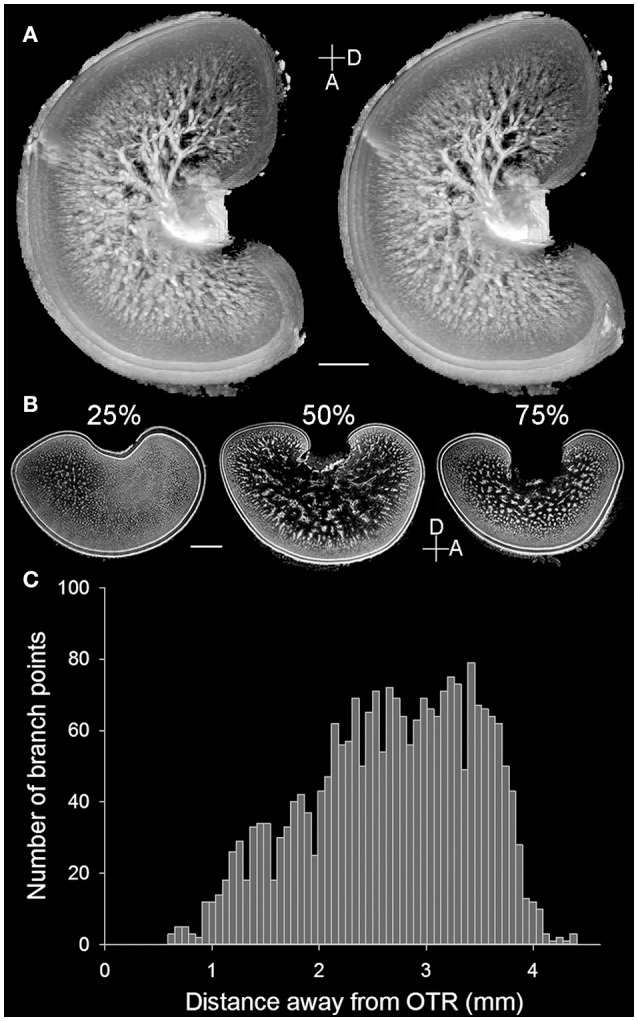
Cell islands in the medulla of the optic lobe are a continuous structure. (A) A pair of stereo images enhanced and reconstructed from the MRI scan showing the 3D structure of cell islands (light areas) in the medulla of the left optic lobe of a cuttlefish (ML = 16 cm). Note that the small opening on the left side of the optic lobe was an artifact caused by the electrode penetration from a separate study. (B) Three cross cryosections at different percentages from the lateral to the medial side of the right optic lobe of a cuttlefish (ML = 19.5 cm) showing the distinct morphologies of the cell islands. D, dorsal; A, anterior. Scale bar, 2 mm. (C) The branch point numbers of the 3D structure of cell islands shown in (A) increase steadily as the distance from the optic tract region (OTR) increases.
